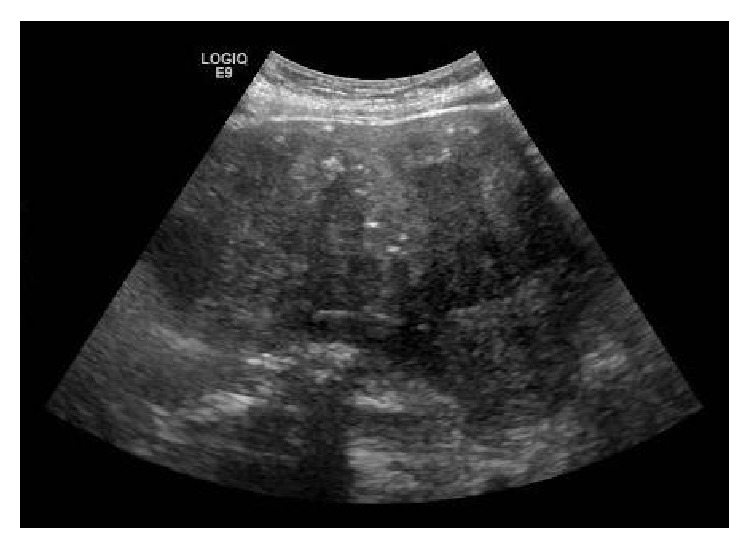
Ultrasound image showing a large pelvic mass.
